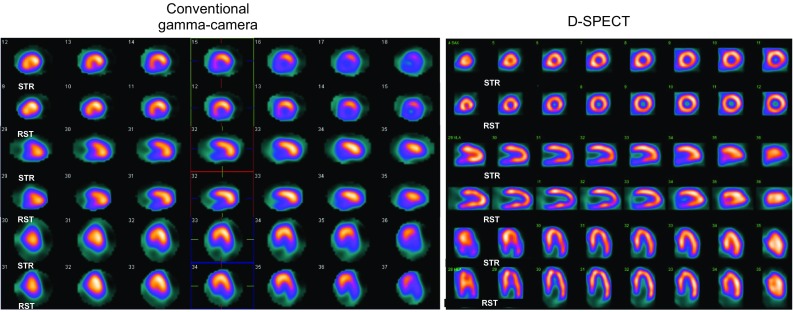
Comparison between stress and rest MPS images of an obese 56-year-old man (BMI 35.5 kg/m^2^) with suspected CAD. The stress images acquired with a conventional dual-head GP gamma camera show a fixed inferior wall perfusion defect due to diaphragmatic attenuation (*left*), while the stress/rest images with a dedicated CZT gamma camera (D-SPECT) and upright patient position show normal perfusion (*right*)
